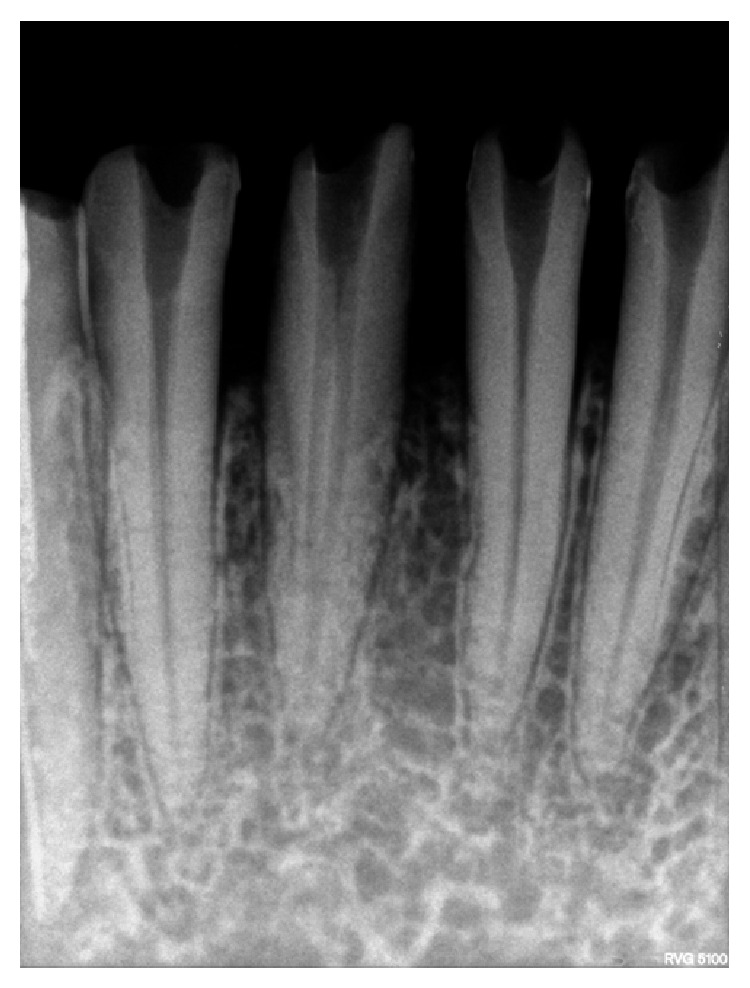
Radiograph showing modified access opening.
